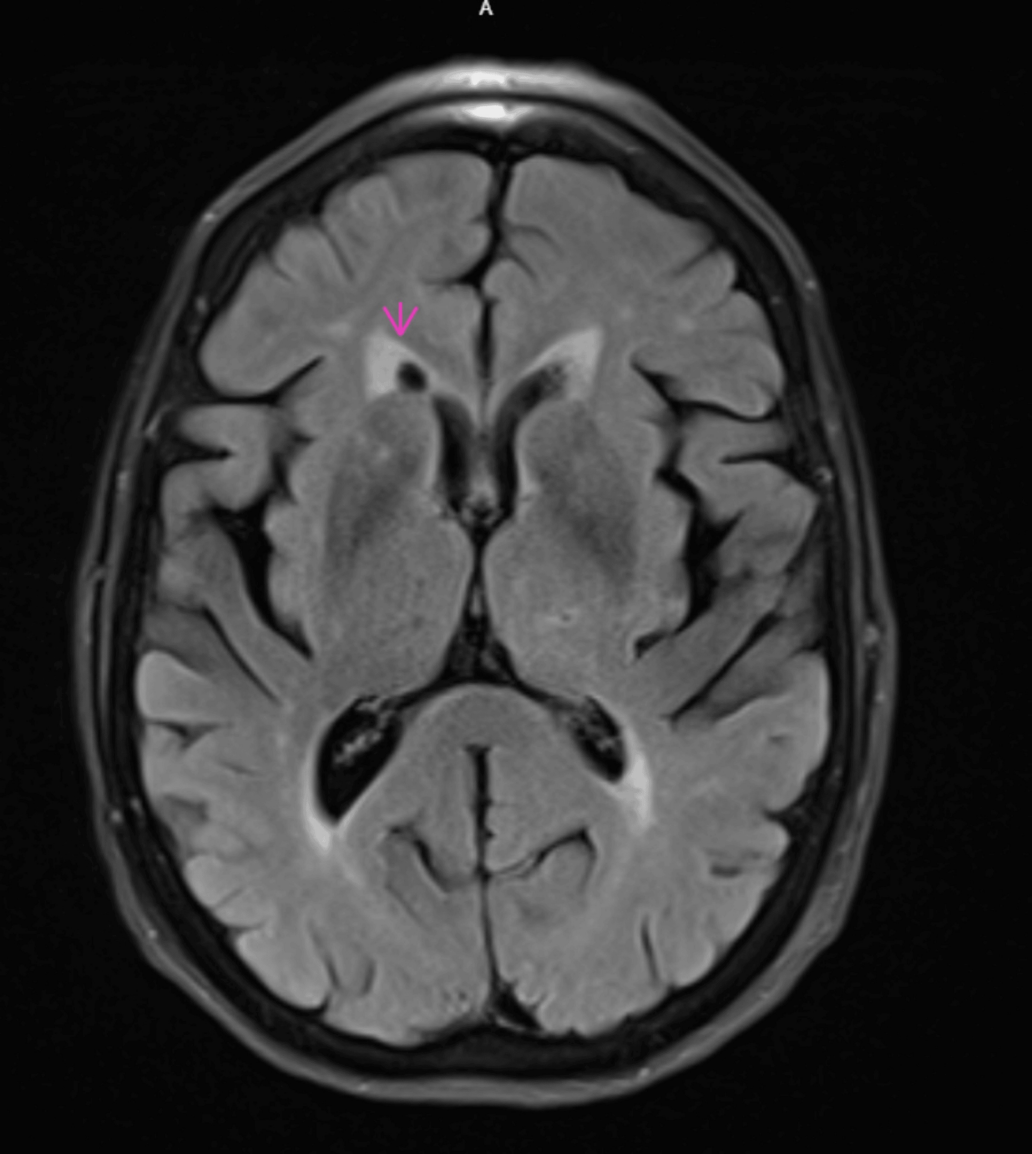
Axial T2 FLAIR MRI of the brain without contrast. FLAIR: fluid-attenuated inversion recovery.
